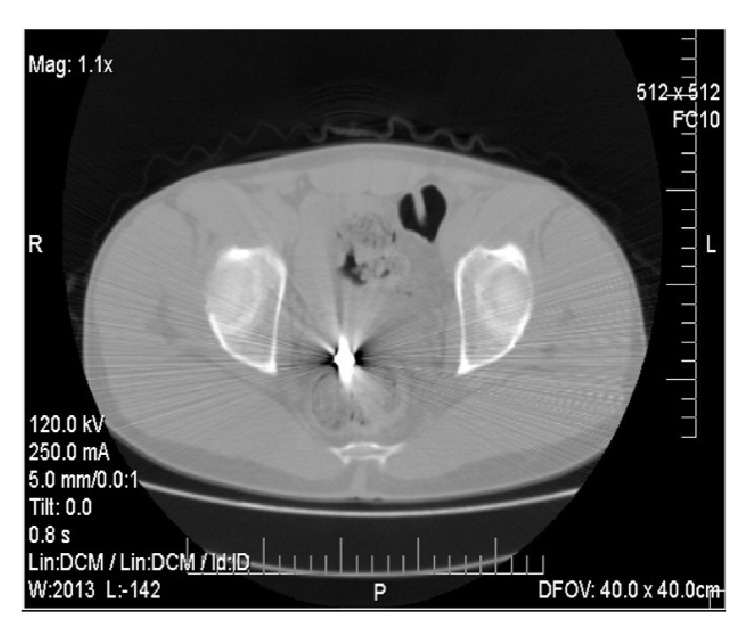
CT scan shows the bullet clear in the lower abdomen.
